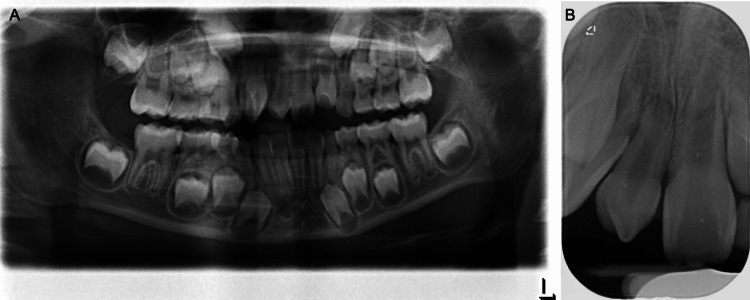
Pre-treatment radiographs. (A) Dental panoramic radiograph, (B) periapical radiograph.
